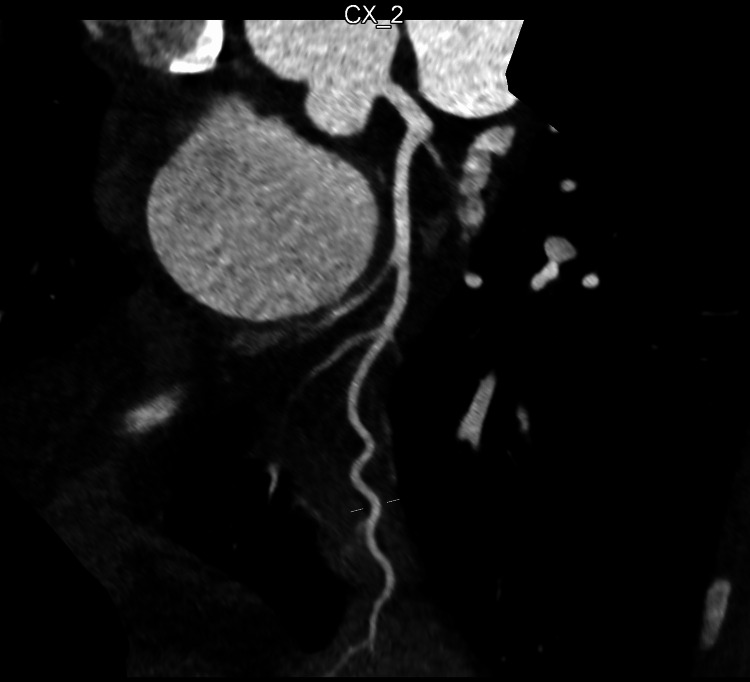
CT coronary angiogram showing left circumflex coronary artery. Normal left circumflex artery with no evidence of coronary atherosclerosis.
